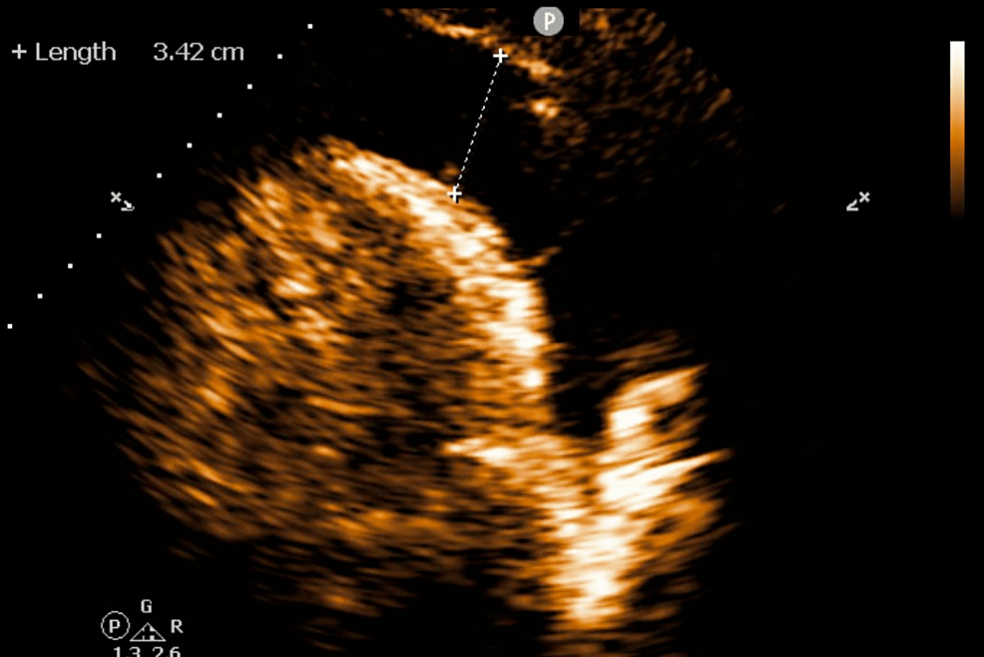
Transthoracic echocardiogram The echocardiogram in the Parasternal Long Axis View (PLAX) is showing a large pericardial effusion.
